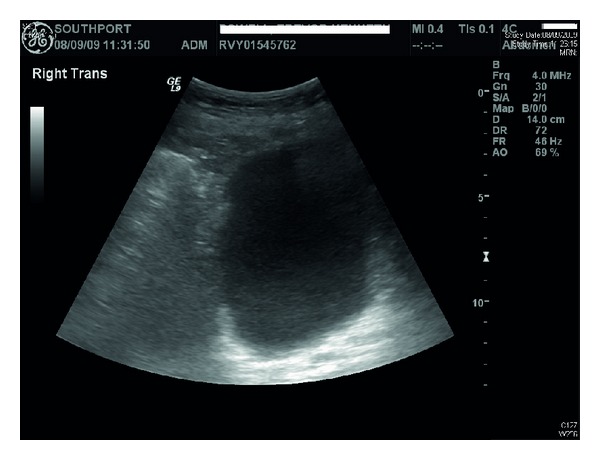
Ultrasound showed trabeculated bladder outline. There was no bladder mass.
